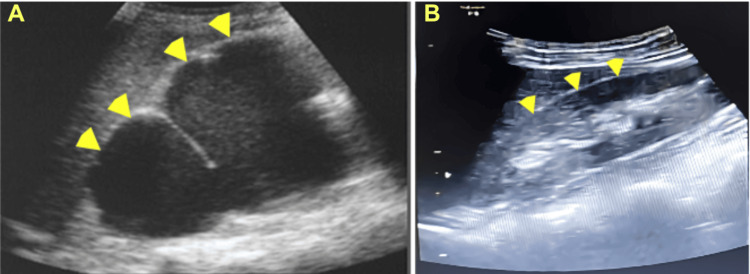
Transabdominal ultrasound findings: (A) Hydronephrosis of the left kidney upon presentation (yellow arrowheads); (B) Resolution of left hydronephrosis after three months of goserelin therapy (yellow arrowheads)
